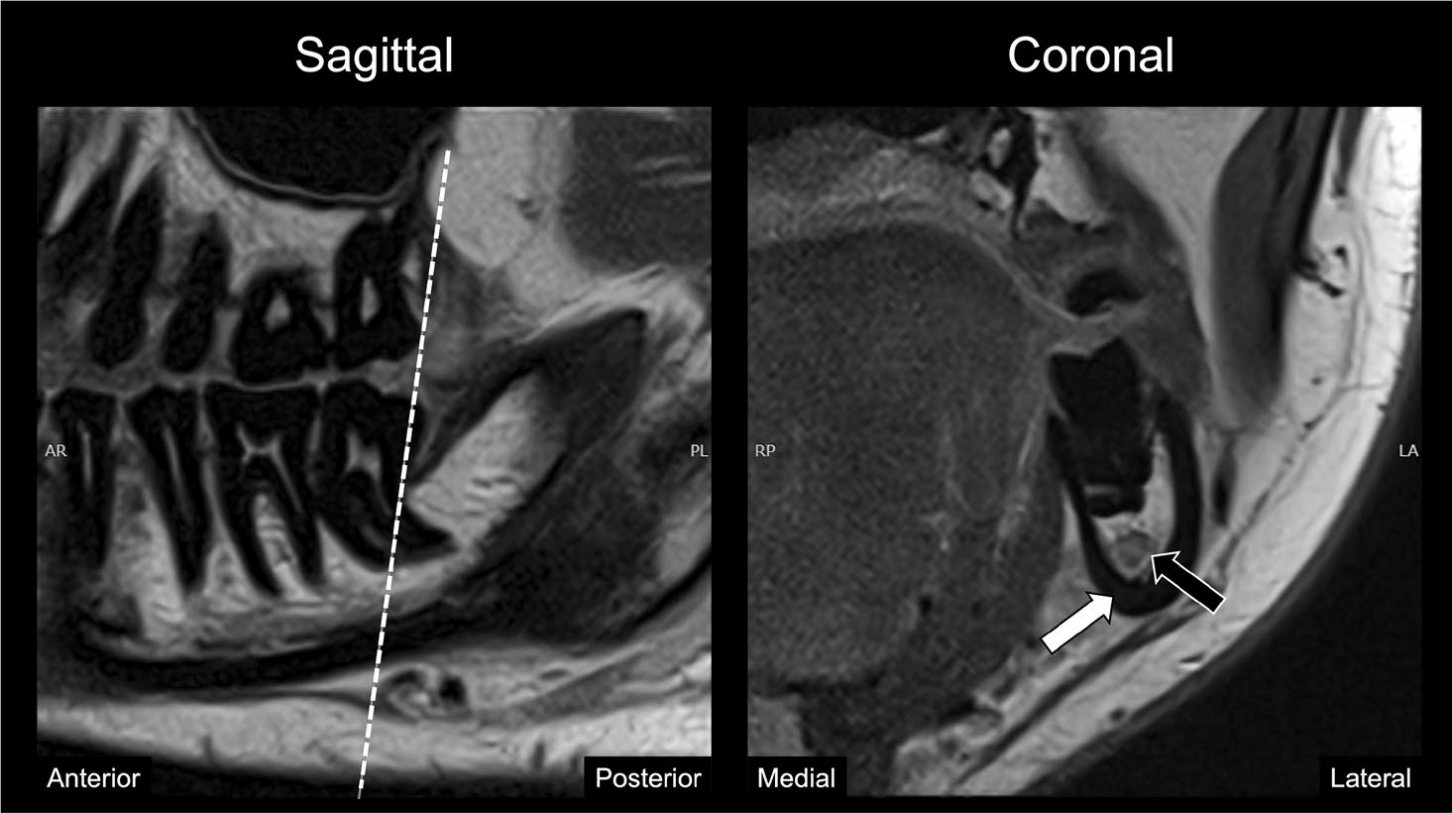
Sagittal and coronal proton‐density‐weighted magnetic resonance images (Siemens MAGNETOM Free. Max 0.55 T; Siemens Healthineers, Forchheim, Germany) acquired with a 7‐channel radiofrequency dental‐dedicated receive coil (RAPID Biomedical GmbH, Rimpar, Germany; resolution: 0.3 × 0.3 × 2 mm; acquisition time: 2′38″; repetition time: 2400 ms; echo time: 48 ms; flip angle: 150°; bandwidth: 100 Hz/pixel; GRAPPA acceleration: × 3, averages: 4) demonstrating the posterior region of the jaws. The images reveal a hyposignal of the teeth and cortical bone (white arrow), as well as a soft tissue‐like signal associated with the dental pulp and mandibular canal (black arrow). The dashed line delineates the anatomical plane from which the coronal section was acquired. Courtesy of Prof. Dr. Rubens Spin‐Neto, Aarhus University, Denmark.
